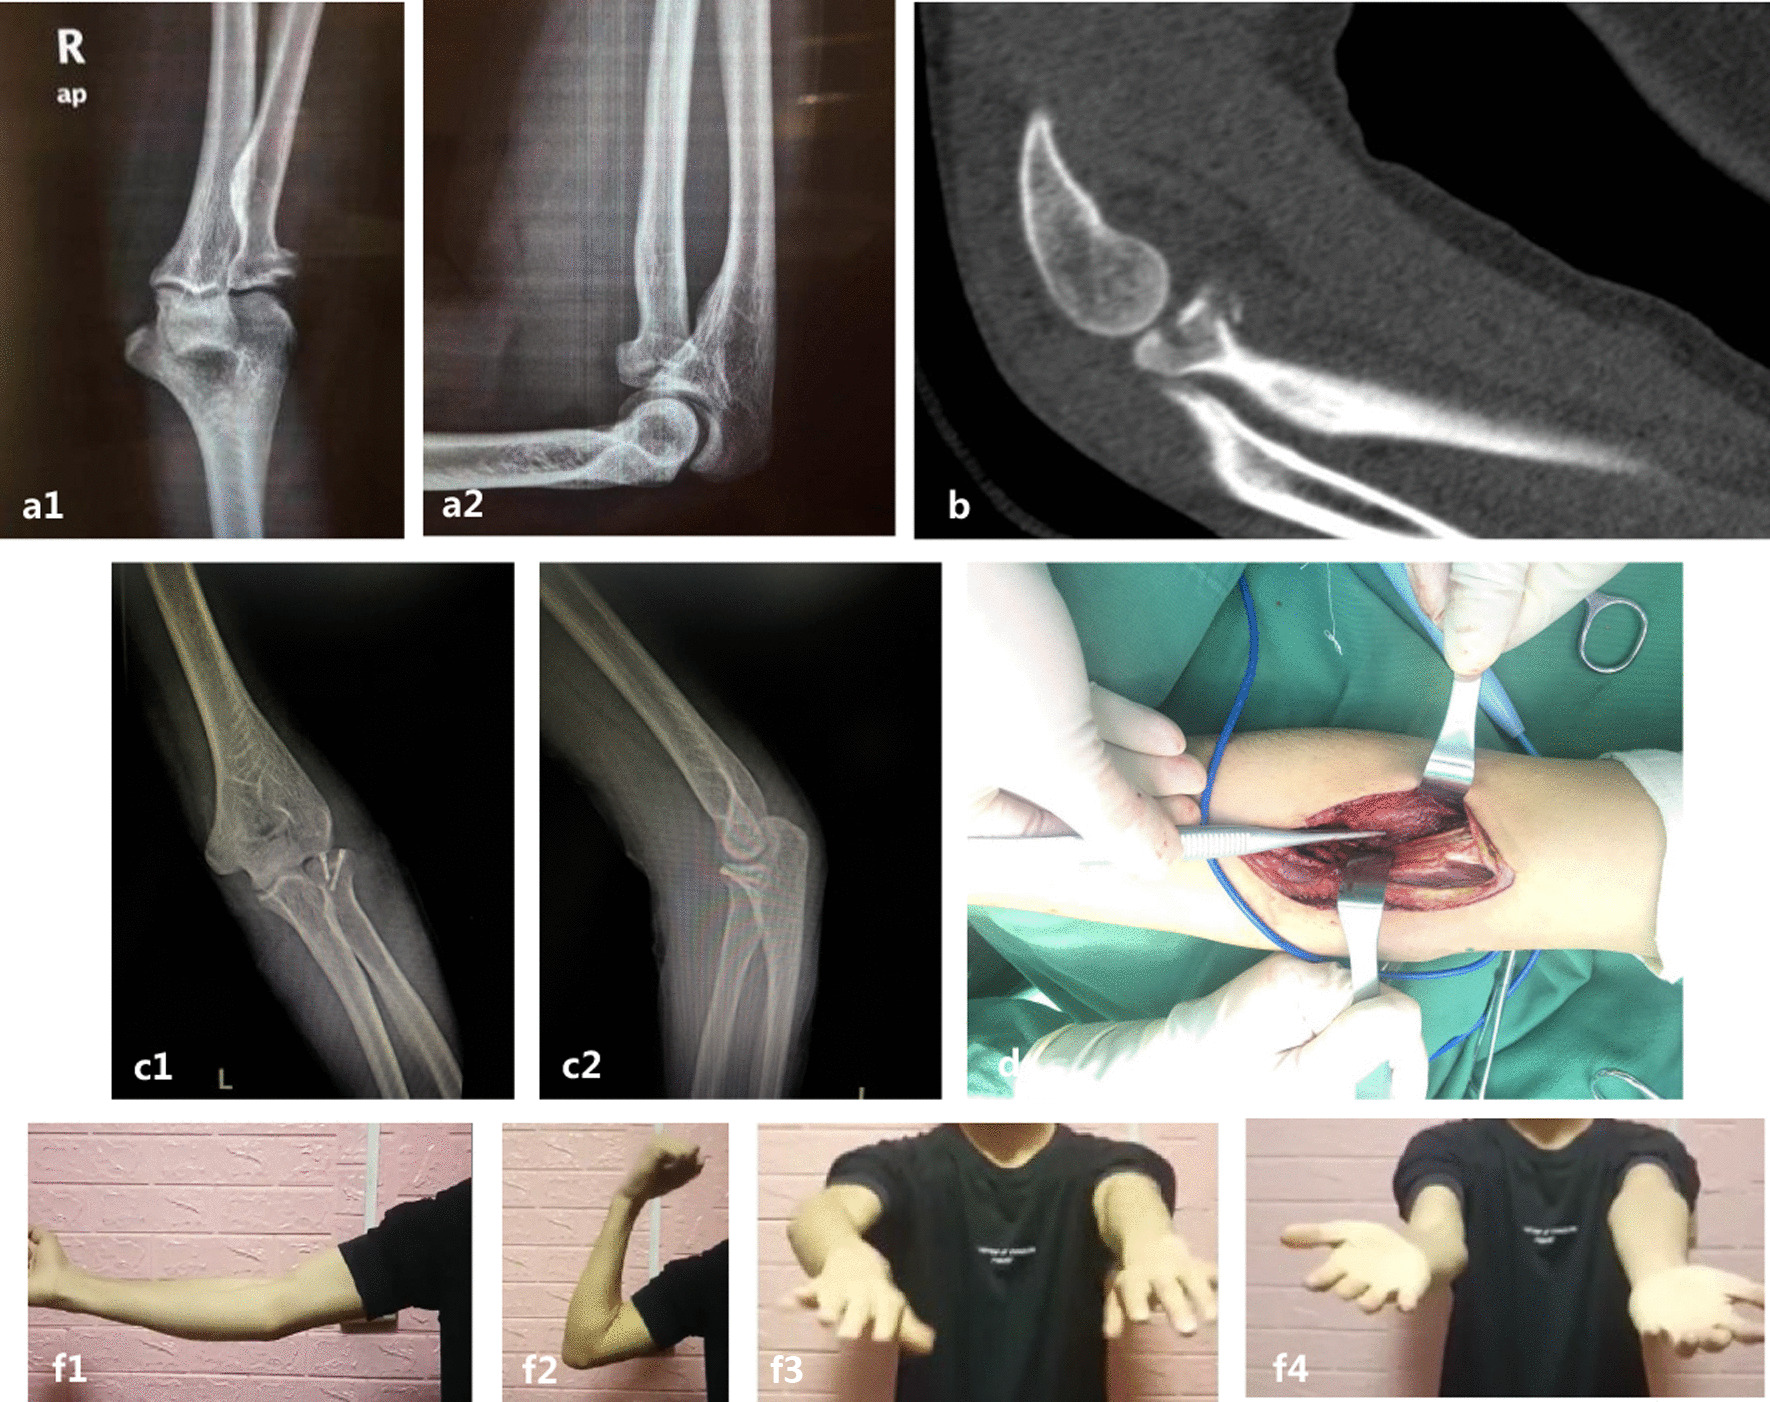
A 23-year-old man presented with a type of mason: II radial head fracture. Preoperative X-ray (a) and computed tomography (b). Intraoperatively, the biceps brachii was exposed and pulled medially to enter between the brachioradialis and biceps brachii interval, with the supinator muscle was located in its deeper layers (d). Solid union and good outcomes were achieved at the 1-year follow-up (c, f)
